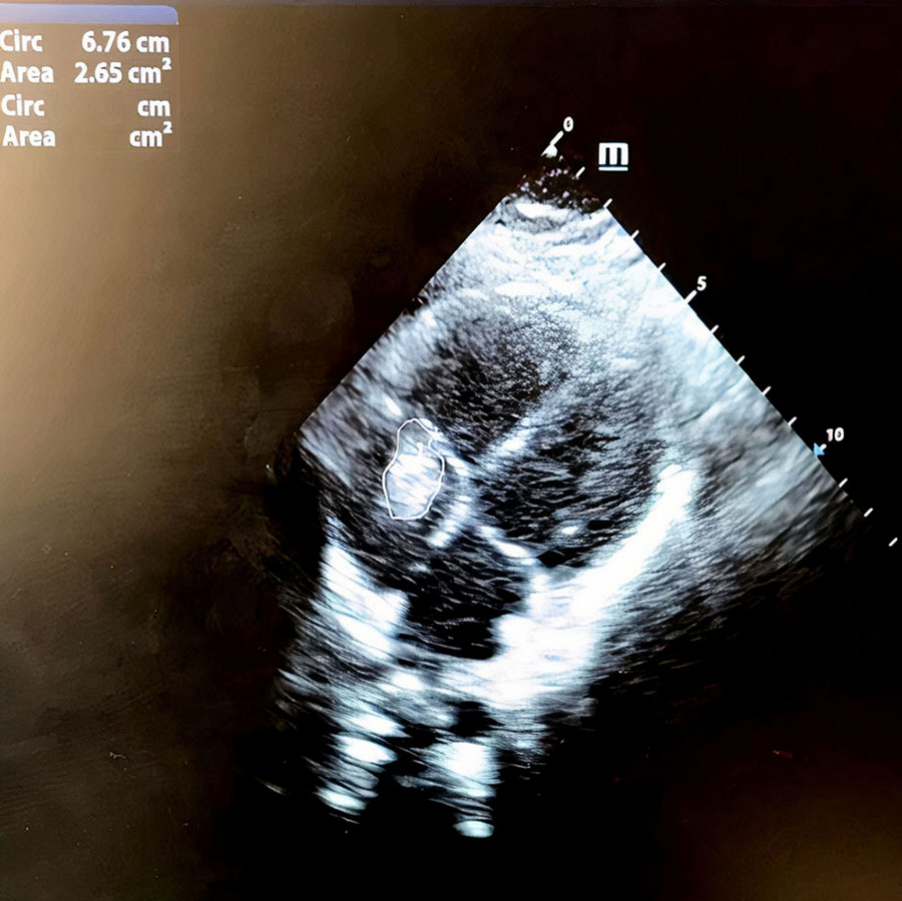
Transthoracic echocardiogram showing a large 2.5 × 1.5 cm mass on tricuspid valve leaflet protruded to the right atrium and right ventricle.
